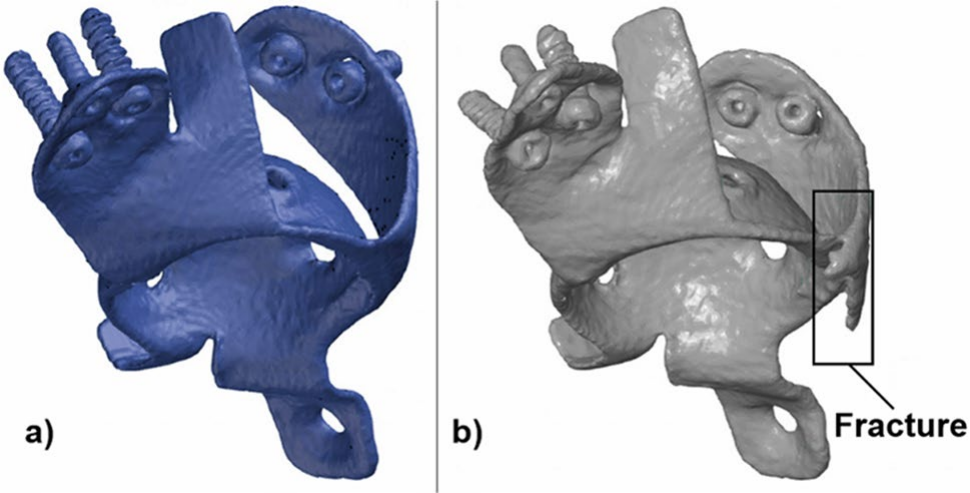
Fracture can be detected investigating the segmented STL files of the acetabular cage from the postoperative CT (a) and from the 1-year control CT (b)
